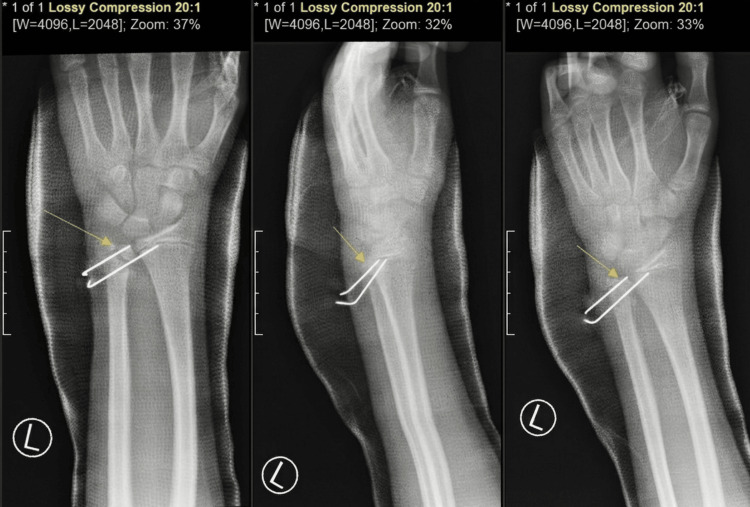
Radiographs at the two-week postoperative follow-up Radiographs of left wrist anteroposterior/posteroanterior (AP/PA; left panel), lateral (middle panel), and oblique (right panel), demonstrating stable Kirschner wire position and no obvious interval loss of alignment on the available view (Day 14).
